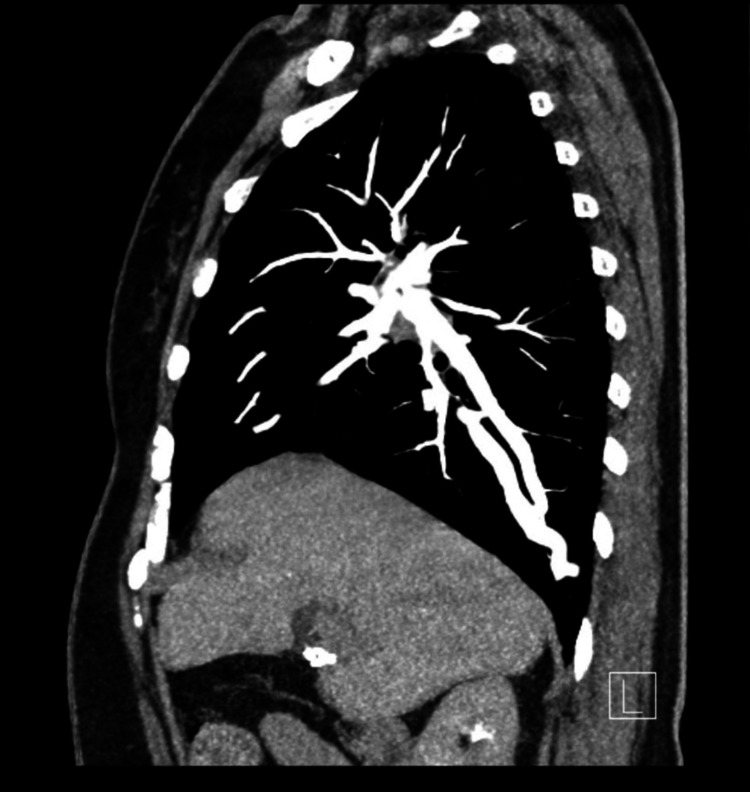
CT angiography revealed a right lower lobe PAVM. PAVM: pulmonary arteriovenous malformations
